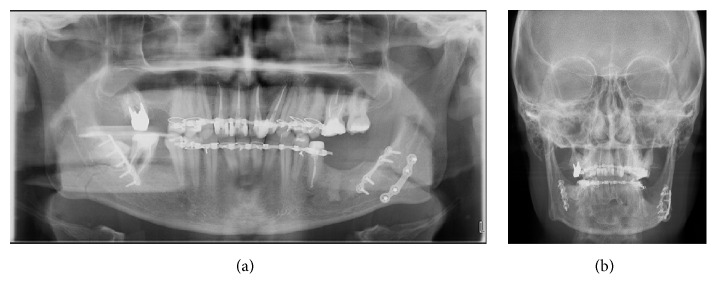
(a) Postoperative panoramic radiograph showing result. (b) Posteroanterior radiograph showing plates configuration and result.
